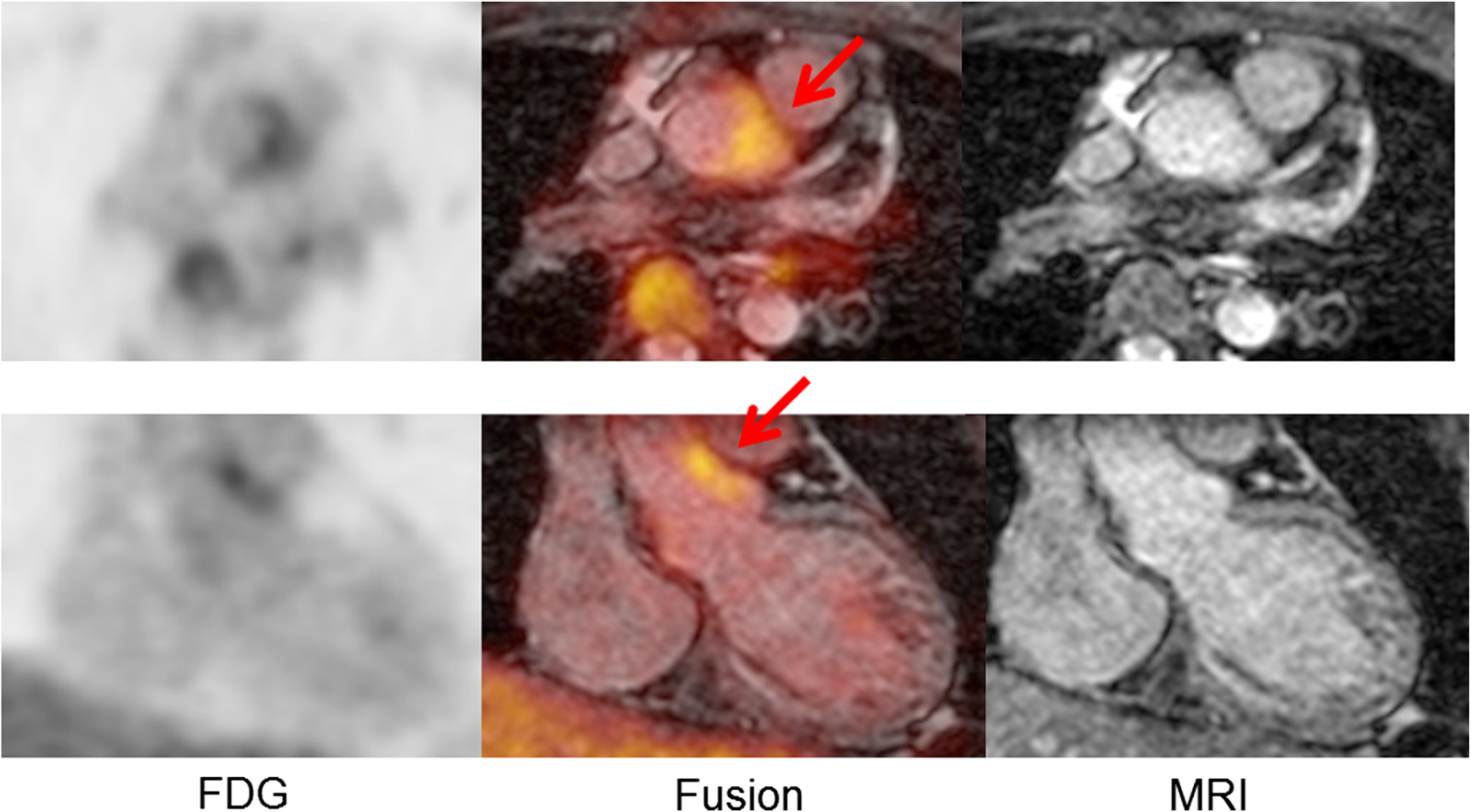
^18^F-FDG PET/MRI images of a patient with Takayasu arteritis. Arrows indicate FDG uptakes on the aortic root, suggesting active vasculitis in the aortic wall and coronary ostial lesions
